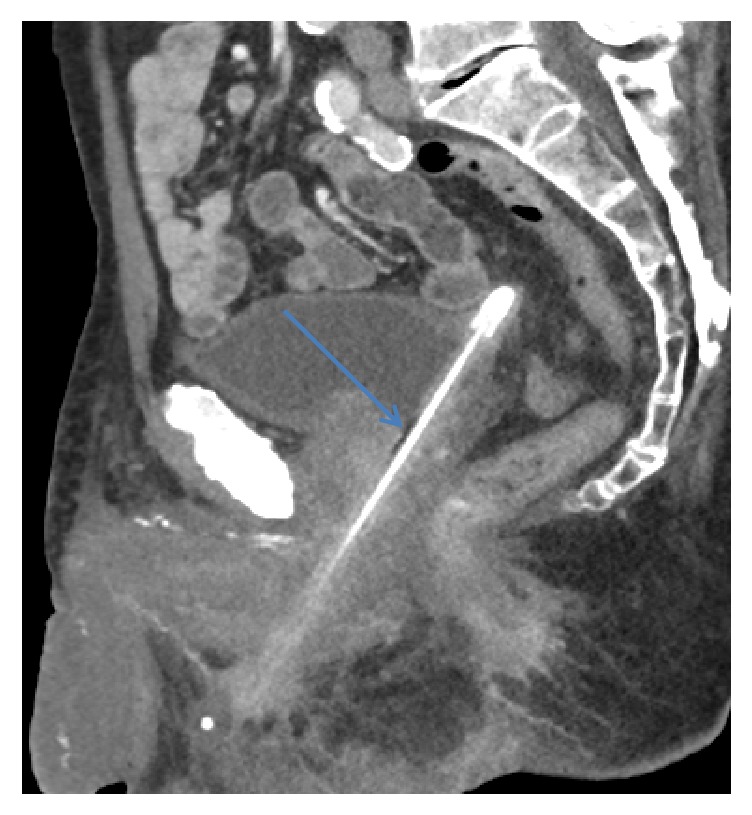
Sagittal images from CT pelvis showing pencil extending from the bladder dome (which is tented superiorly) through the prostatic urethra then through a perforation in the bulbar urethra.
